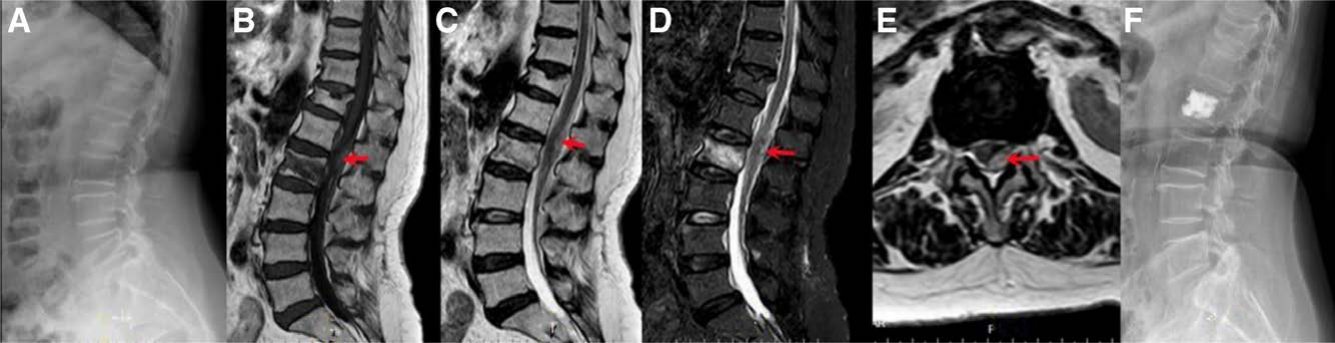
(A) X-rays showed vertebral compression fractures at T12 and L2. (B–E) MRI showed acute compression fracture of L2 body and epidural hematoma extending from L2 to L1. (F) X-rays showed that bone cement was well localized in the L2 vertebral body. MRI = magnetic resonance imaging.
